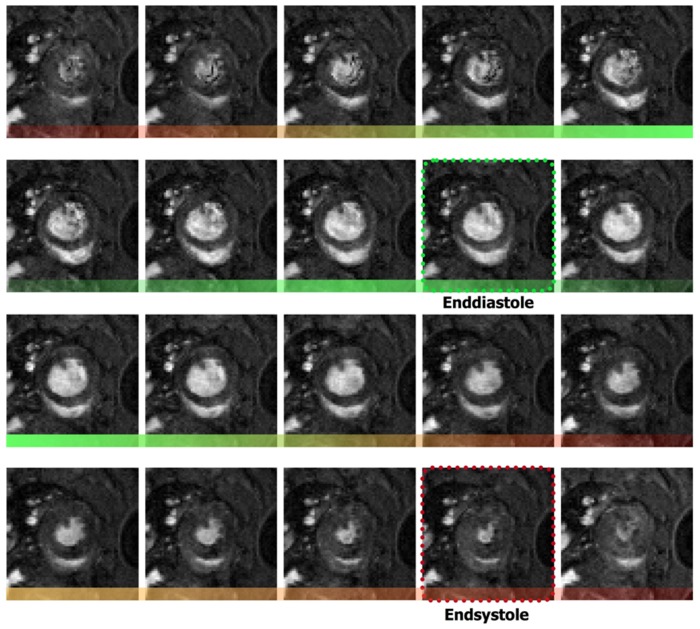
Representative full cycle of a cardiac short-axis cine-MRI with 20 frames.
